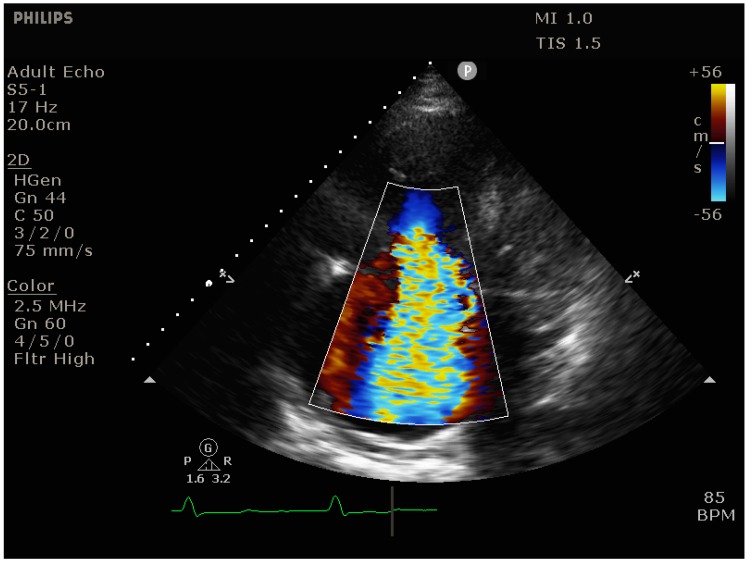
Transthoracic echocardiogram (apical 4-chamber view) showing severe tricuspid regurgitation due to massive RV dilation.
